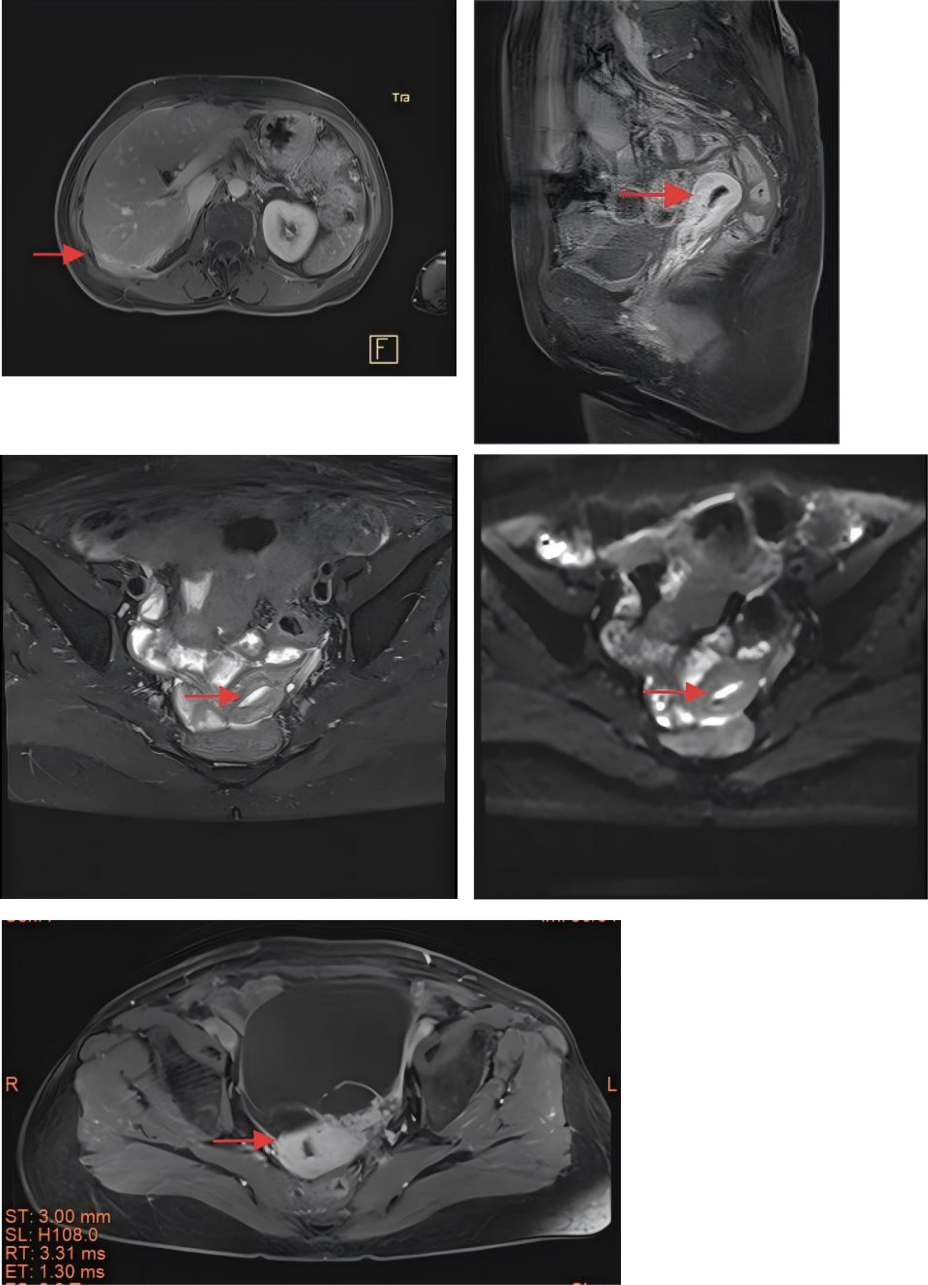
Metastatic tumors of the liver and uterus. ① is a liver metastatic tumor; ②, ③, and ④ are endometrial metastatic tumors, with the endometrium thickened in a ring shape. DWI shows diffusion limitation and significant delayed enhancement on the enhanced scan; ⑤ is a metastatic giant tumor of the uterus and ovary.
